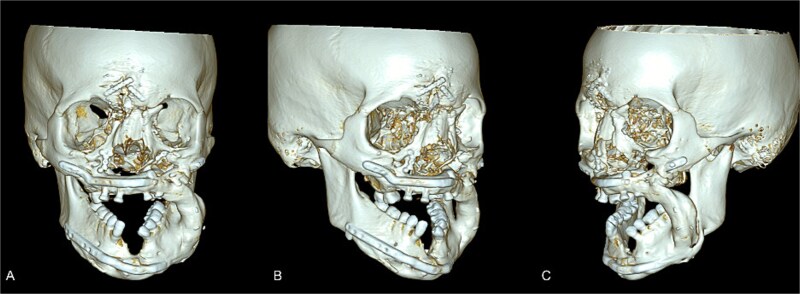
Maxillofacial CT with 3-D volume after fibula free flap. The reconstructed left midface/mandible with fibula free-flap hardware is shown with resection margins and the osseous bridge. (A) Frontal view. (B) Right anterior view. (C) Left anterior view.
